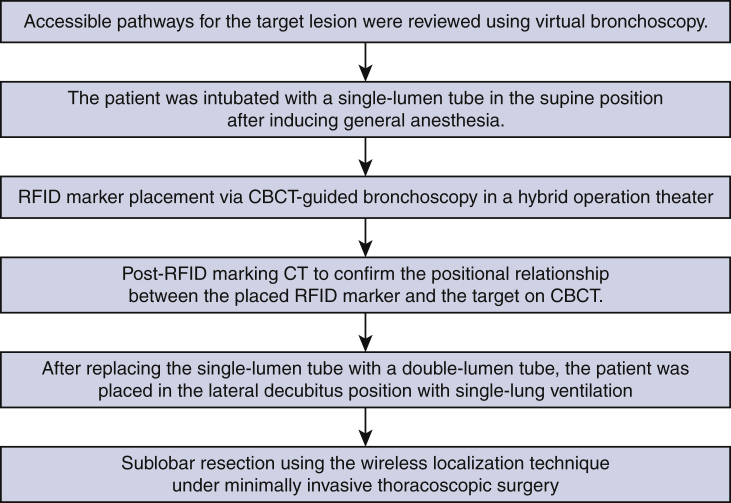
Steps in the radiofrequency identification (*RFID*) marking procedure. *CBCT*, Cone-beam computed tomography; *CT*, computed tomography.
